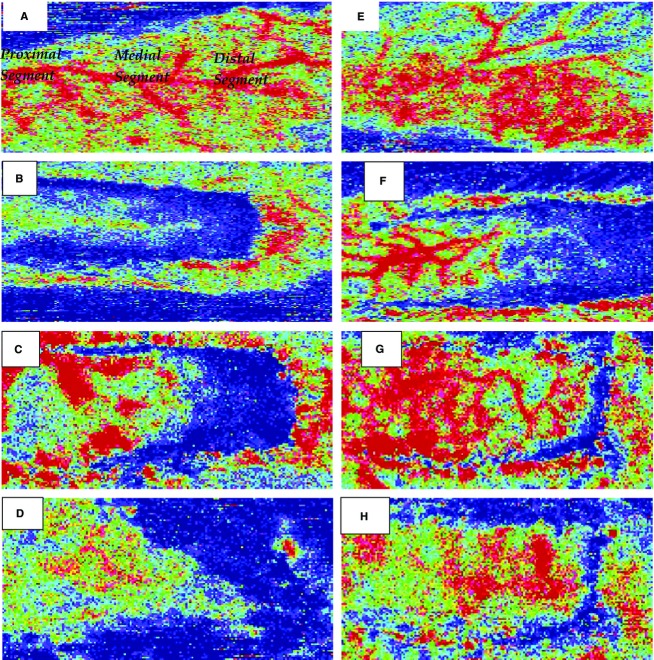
Representative Laser Doppler image of blood flow and its return to the dorsal surface of skin flap wounds treated with 0.9% saline (A through D) or nsPRP (E through H). The blue color within the 3 X 8 wound area is indicative of a lack of blood flow. The red color indicates the presence of blood flow.
